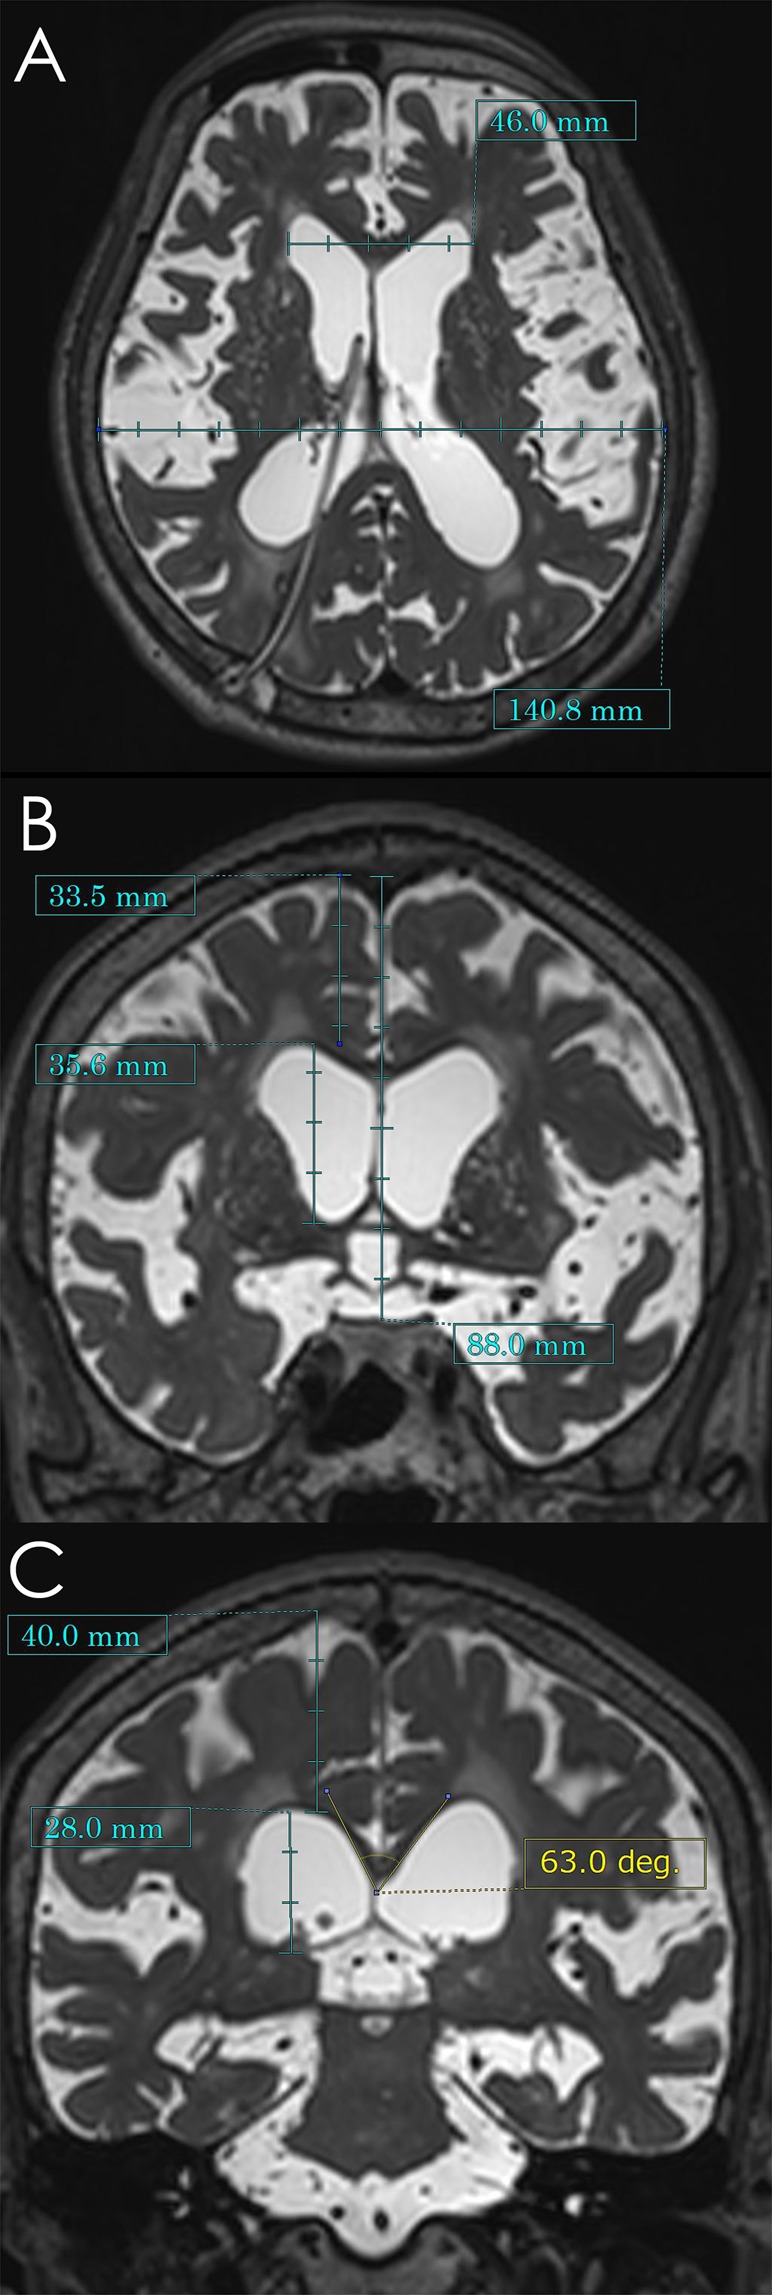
Morphological indices specific to idiopathic normal pressure hydrocephalus (iNPH). These magnetic resonance images are shown for the same patient as Fig. 6. The Evans index was calculated as 0.327 (A); the maximum length of the frontal horns of the lateral ventricles (46.0 mm) divided by the maximum cranial length on the same axial plane (140.8 mm). The z-Evans index, which was calculated as the maximum z-axial length of the frontal horns of the lateral ventricles (35.6 mm) divided by the maximum cranial z-axial length at the midline (88.0 mm) on the coronal plane just on the anterior commissure (AC), was 0.405 (B). The callosal angle was calculated as 63 degrees (C) at the roof of the bilateral ventricles on the coronal plane just on the posterior commissure (PC). The brain per ventricle ratios (BVRs) at the AC and PC levels were calculated as 0.941 (B) and 1.429 (C), respectively.
